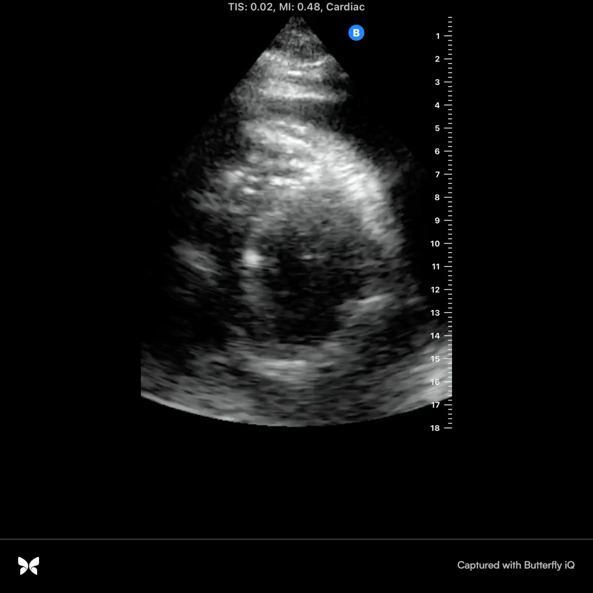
Parasternal short axis view by point of care cardiac ultrasonography revealing moderate to large pericardial effusion.
